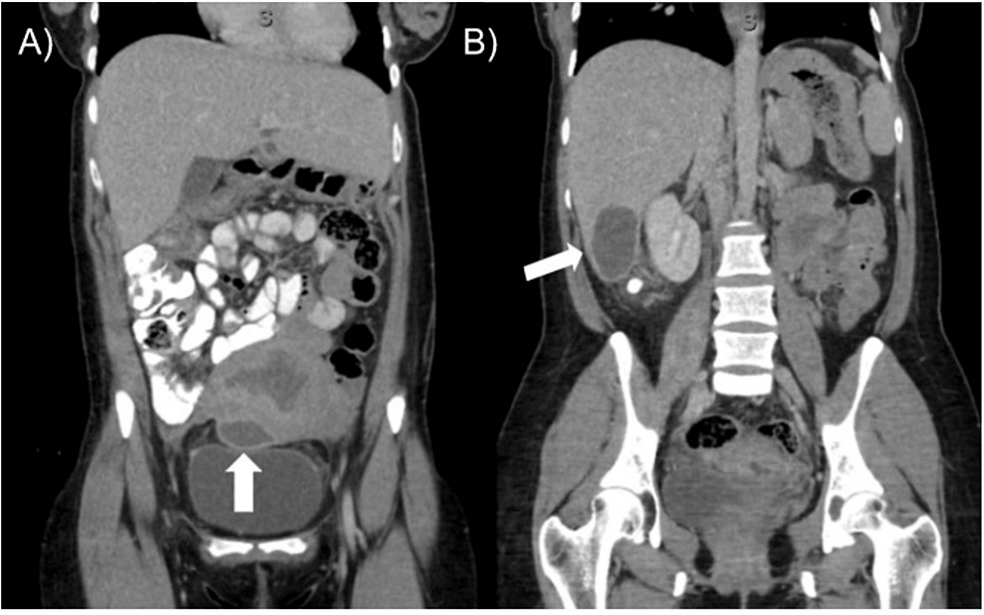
Computed tomography imaging reveals periuterine and subhepatic abscesses A) Coronal computed tomography (CT) scan from the patient’s presentation to the emergency room on postoperative day 12 showing an enlarged uterus measuring 14.3 x 10.3 x 7.1 cm with a 4.4 cm rim-enhancing, high-density cystic structure near the serosal surface of the anterior uterus. B) Coronal CT showing high-density 5.5 cm fluid collection on the inferior margin of the right lobe of the liver.
